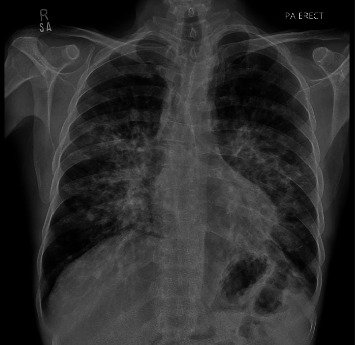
Chest X-ray (PA erect) showing bilateral perihilar nodularity, more pronounced in the infrahilar region. Given the history of Kaposi sarcoma, findings are suggestive of metastatic deposits, with atypical bacterial infection as a differential consideration.
